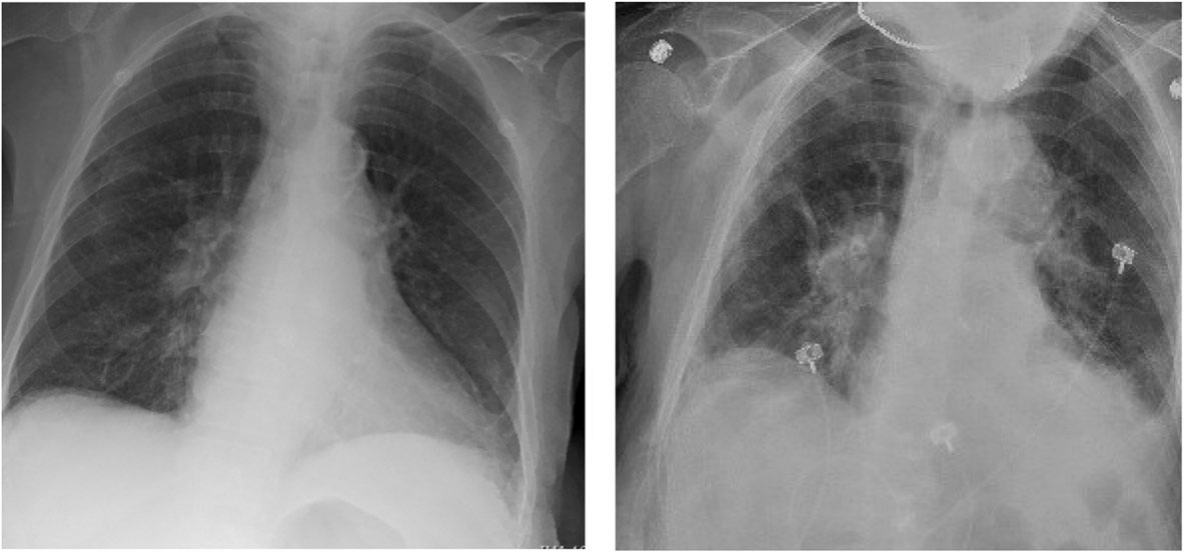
Chest radiographs. Chest X-ray on admission (left) and on hospital day 13 (right), showing worsening bilateral infiltrates
